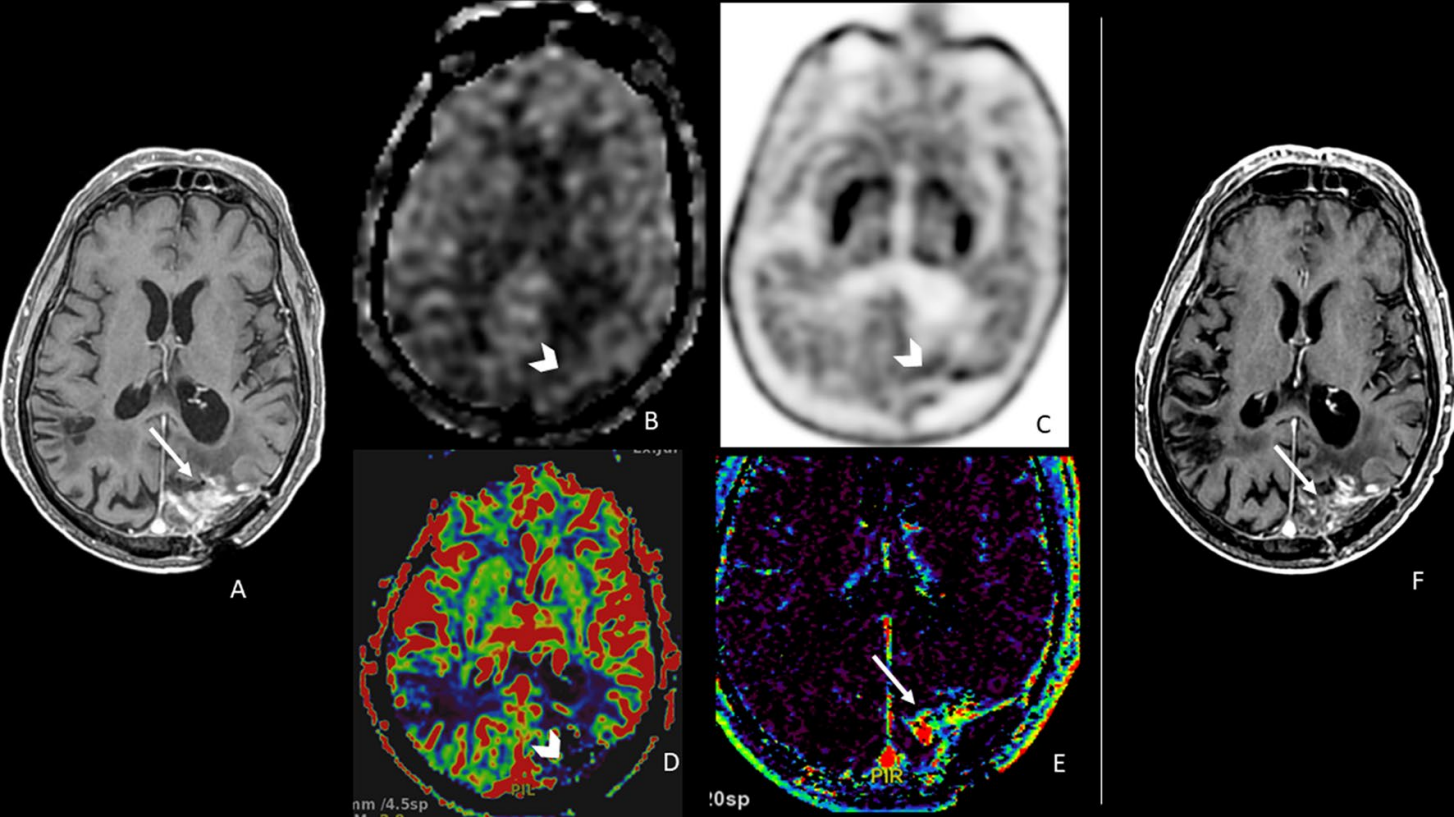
A representative case of a high-grade glioma with an enhancing lesion in the left parietal lobe (a, arrow) occurring 8 months after radiation therapy. The lesion did not show high values of ASL_CBF (b, arrowhead), DSC_rCBV (d, arrowhead), or a significant increase in 18F-DOPA PET uptake (c, arrowhead). DCE_Ve showed foci of increased values (e, arrow). Nine-month MRI follow-up showed the stability of the lesion with a slight reduction in contrast enhancement (f, arrow). The lesion was classified as post-treatment changes, and ASL, DSC, and PET were concordant and negative, whereas DCE_Ve misclassified the lesion. ASL = arterial spin labeling; CBF = cerebral blood flow; DSC = dynamic susceptibility contrast-enhanced perfusion; DCE = dynamic contrast-enhanced perfusion; CBV = cerebral blood volume; Ve = fractional volume of the extravascular extracellular space
